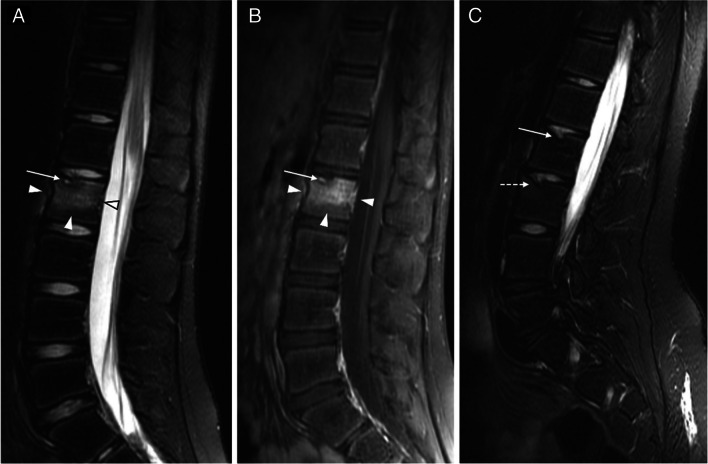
Resolution of inflammatory findings in vertebral lesions: magnetic resonance images of the lumbar spine obtained from a girl at ages 12 (a, b) and 16 (c) years. a, b Sagittal T2-weighted fat-saturated (a) and sagittal T1-weighted contrast-enhanced fat-saturated (b) images of the lumbar spine show bone edema and enhancement of the body of lumbar (L) vertebra 2 (*arrowheads*). There is also a Schmorl node of the superior vertebral endplate of L2 (*arrow*). c Sagittal T2-weighted fat-saturated image of the same patient 4 years later shows the complete resolution of the inflammatory changes of L2, with resolution of the edema. There is a persistent Schmorl node of the superior vertebral endplate of L2 (*arrow*), representing a permanent sequela. Note also another Schmorl node in L3 (*broken arrow*), representing a sequela of another lesion that occurred in the time between the two exams
